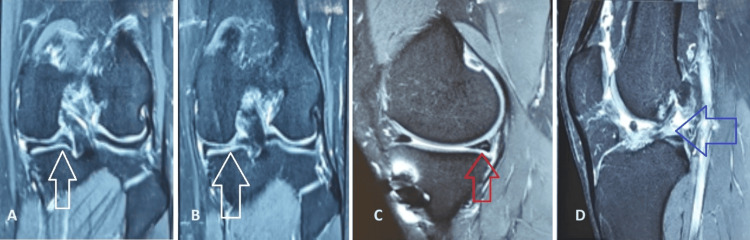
MRI images of the knee A and B: White arrow pointing toward the medial meniscus tear in the coronal section. C: Red arrow pointing toward the posterior horn of the medial meniscus tear in the sagittal section. D: Blue arrow pointing toward a full-thickness ACL graft rupture in the sagittal section. MRI: magnetic resonance imaging, ACL: anterior cruciate ligament
